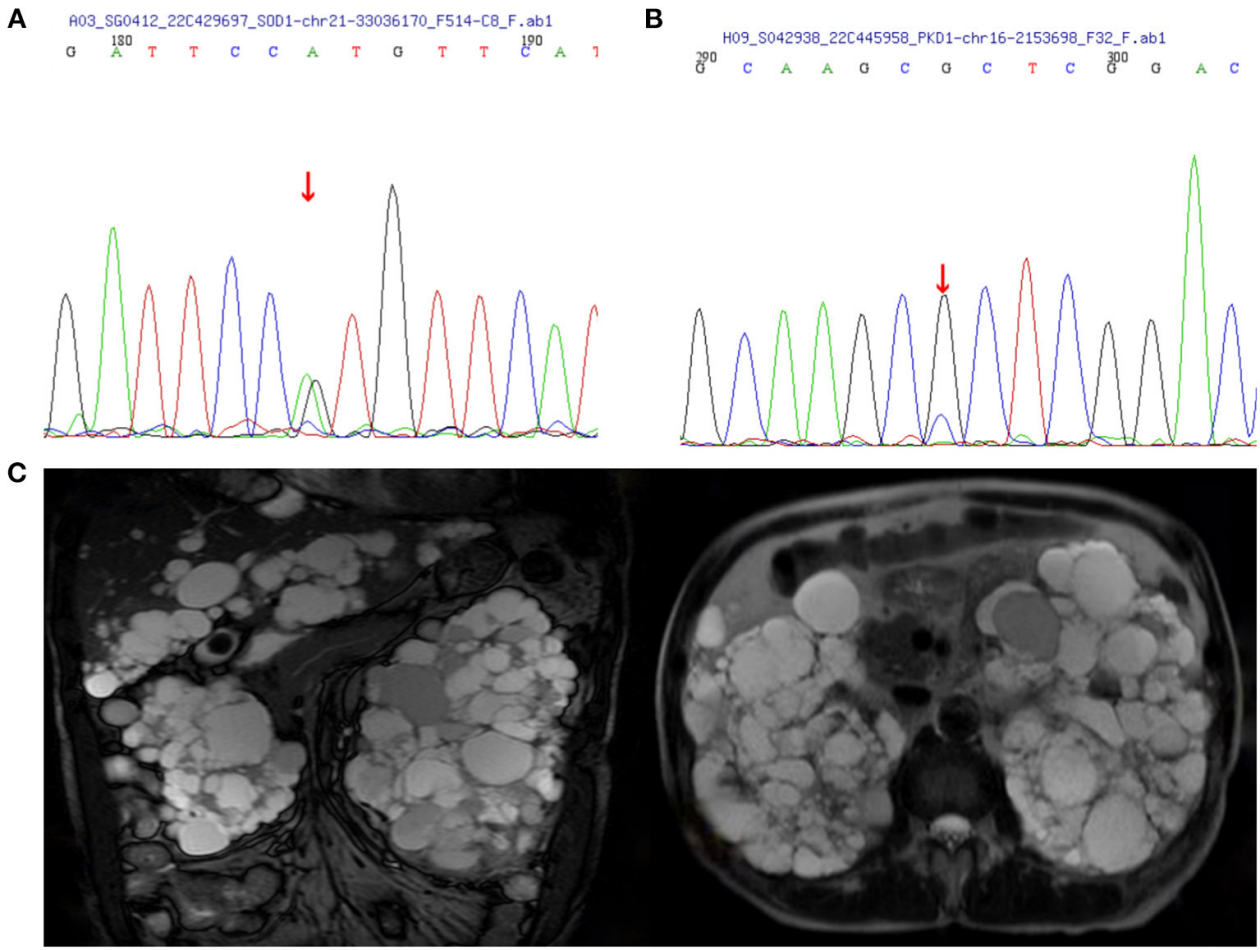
Images of polycystic kidney disease and sanger sequence. (A) SOD1:c.140(exon2)A>G. (B) PKD1:c.8360(exon23)G>C. (C) MRI images of the case IV-3 associated with polycystic liver disease and polycystic kidney disease.
